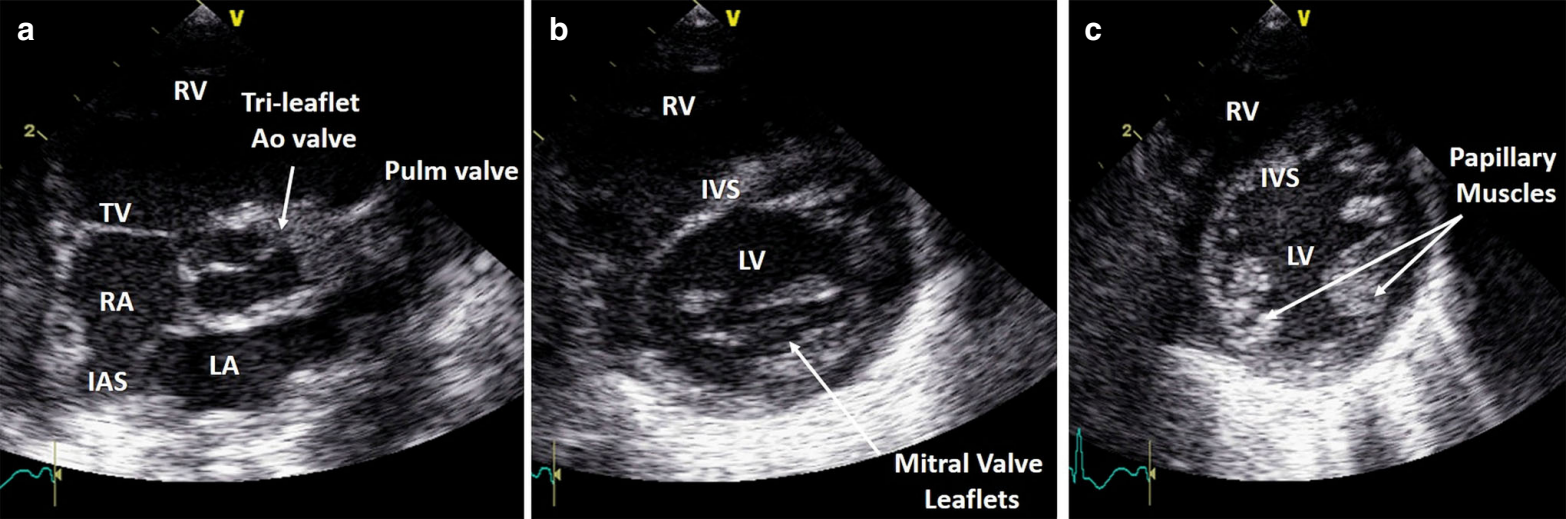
Parasternal short axis view at the level of the aorta (a), mitral valve (b), and papillary muscles (c). Ao aorta, LV left ventricle, LA left atrium, IVS inter-ventricular septum, IAS interatrial septum, RV right ventricle, TV tricuspid valve, IVC inferior vena cava
